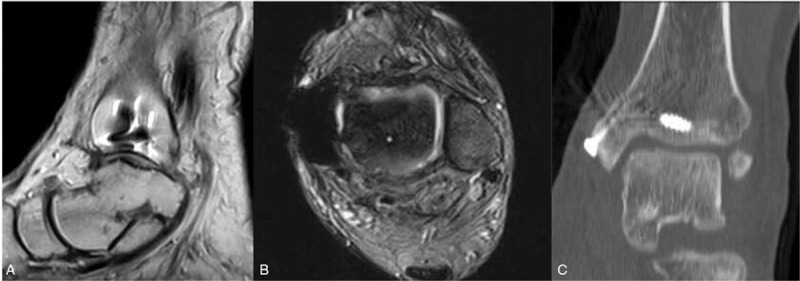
The magnetic resonance (MR) and computed tomography (CT) images of the left ankle joint after 2 years, post-surgery. The sagittal T1-weighted (A) and transverse T2-weighted (B) MRI are shown. The CT image of the left ankle joint is shown (C).
